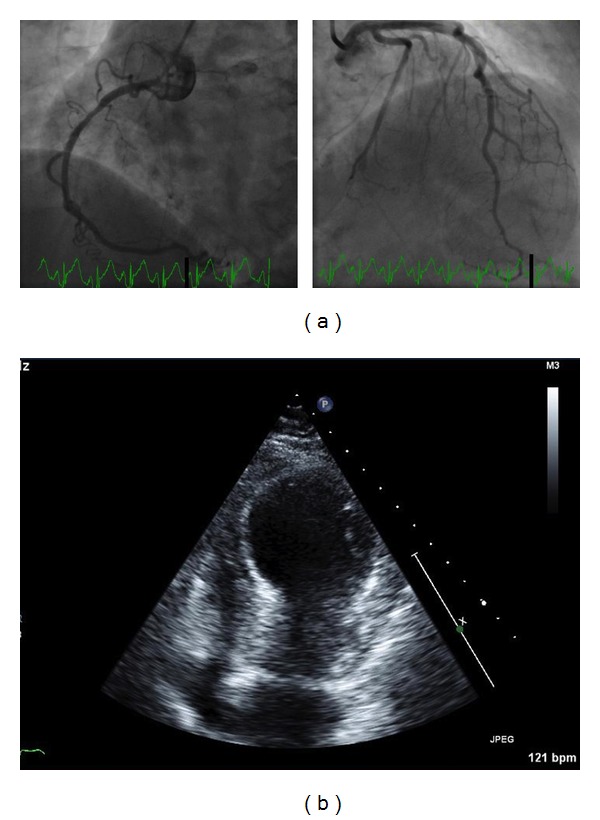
(a) Coronary angiography showing nonobstructing 30% stenosis of right coronary artery with otherwise normal coronary arteries. (b) Transthoracic echocardiogram showing anteroapical and infra-apical wall hypokinesis with ejection fraction of 20%.
